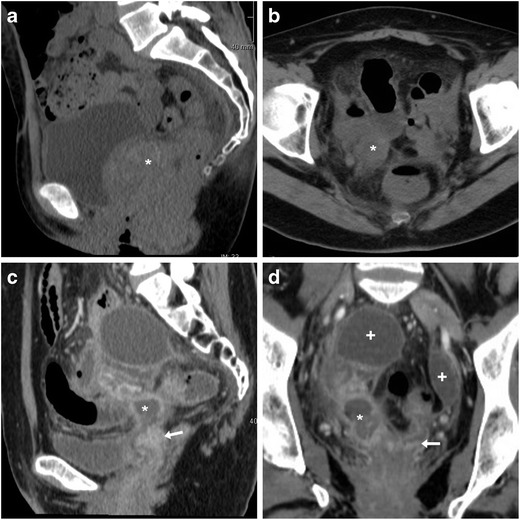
Two cases of vaginal vault haematoma. A) Typical example of midline haematoma after VH for GP in a 40-year-old female: precontrast sagittal CT image showing 5-cm hyperattenuating (45–50 Hounsfield units, HU) mass-like structure (*) above the vaginal dome, causing compression of the bladder. B–D) in a 35-year-old female, following VH for menorrhagia, a smaller (3 × 2 cm) paramedian vaginal vault haematoma (*) shows mild precontrast (B) hyperattenuation (40 HU), peripheral enhancement after iv contrast (C, D), abutting the upper right aspect of the vaginal dome (arrows). Both occurrences were successfully managed conservatively. Note: lymphocele (+). (Partially reproduced from open access ref. no [58])
